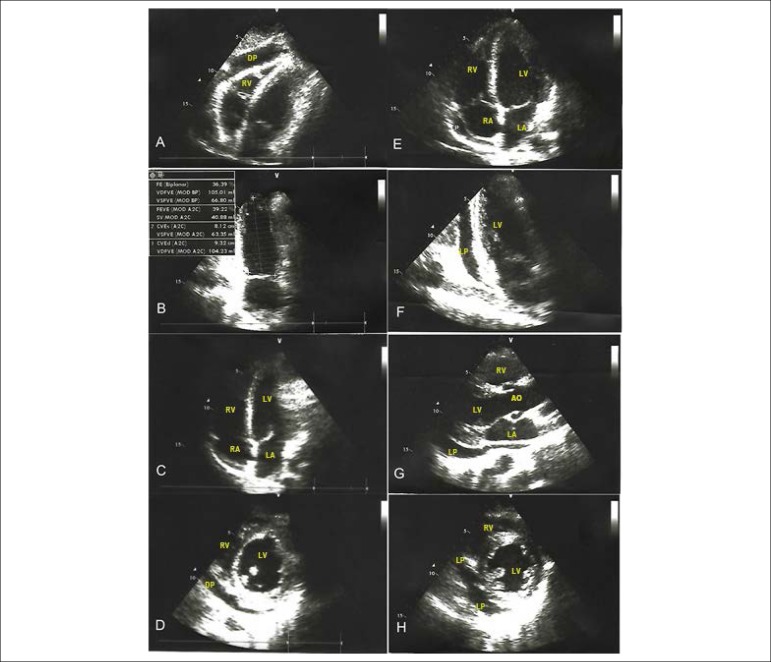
Transthoracic echocardiogram with altered left ventricular ejection fraction (36.39%), left ventricular hypercontractility, and mild bilateral pericardial effusion (A-D). E-H shows a second transthoracic echocardiogram with ejection fraction of 70%. DP and LP: pericardial effusion. RV: right ventricle; LV: left ventricle; RA: right atrium; LA: left atrium; EF: ejection fraction.
